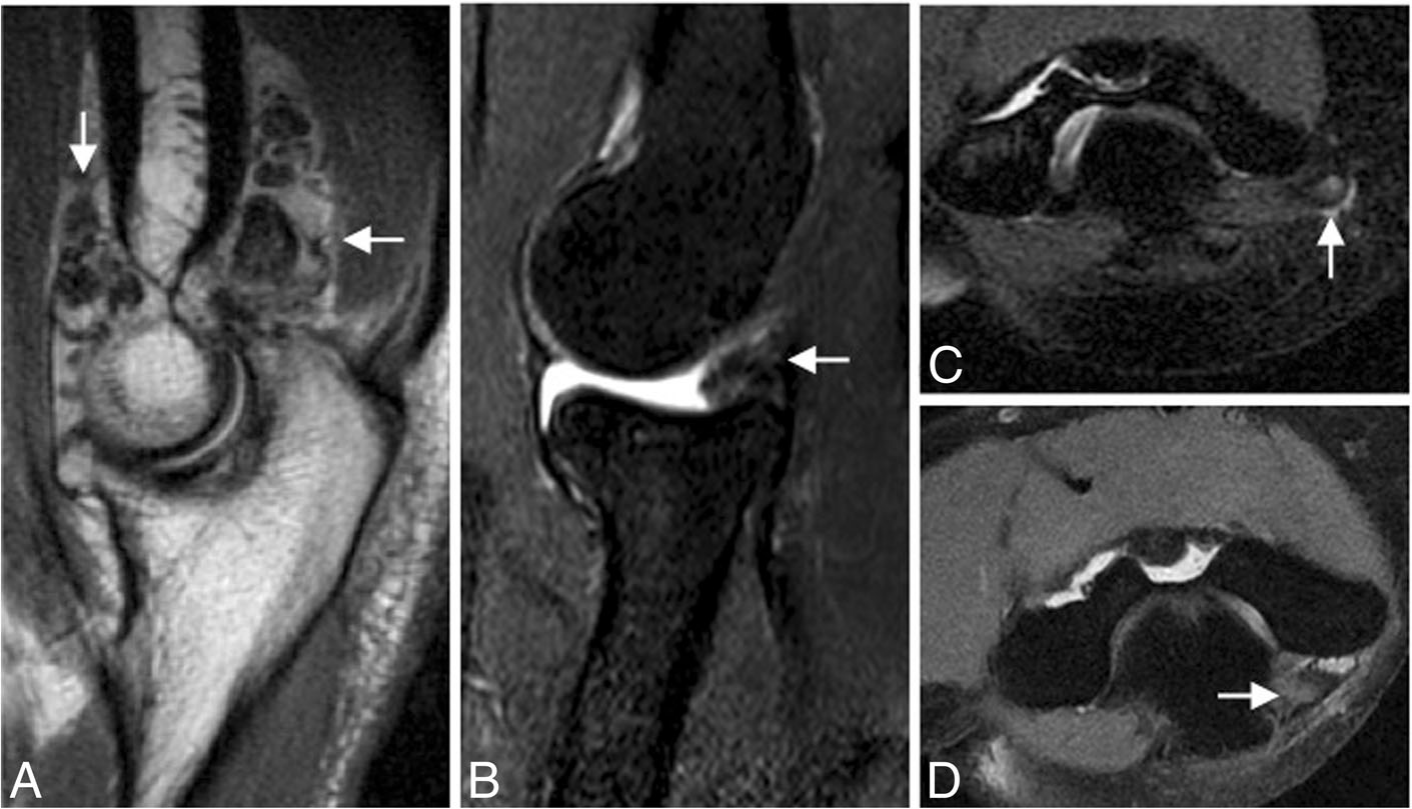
Four different patients with recurrent painful click in the elbow. Sagittal T2-weighted MRI (a), sagittal FS PD-weighted MRI (b), axial FS PD-weighted MRI (c), and axial T1-weighted MRI (d) showing synovial osteochondromatosis (a), a posterolateral plica (b), an ulnar nerve subluxation (c), and hypertrophy of the medial head triceps (d)
